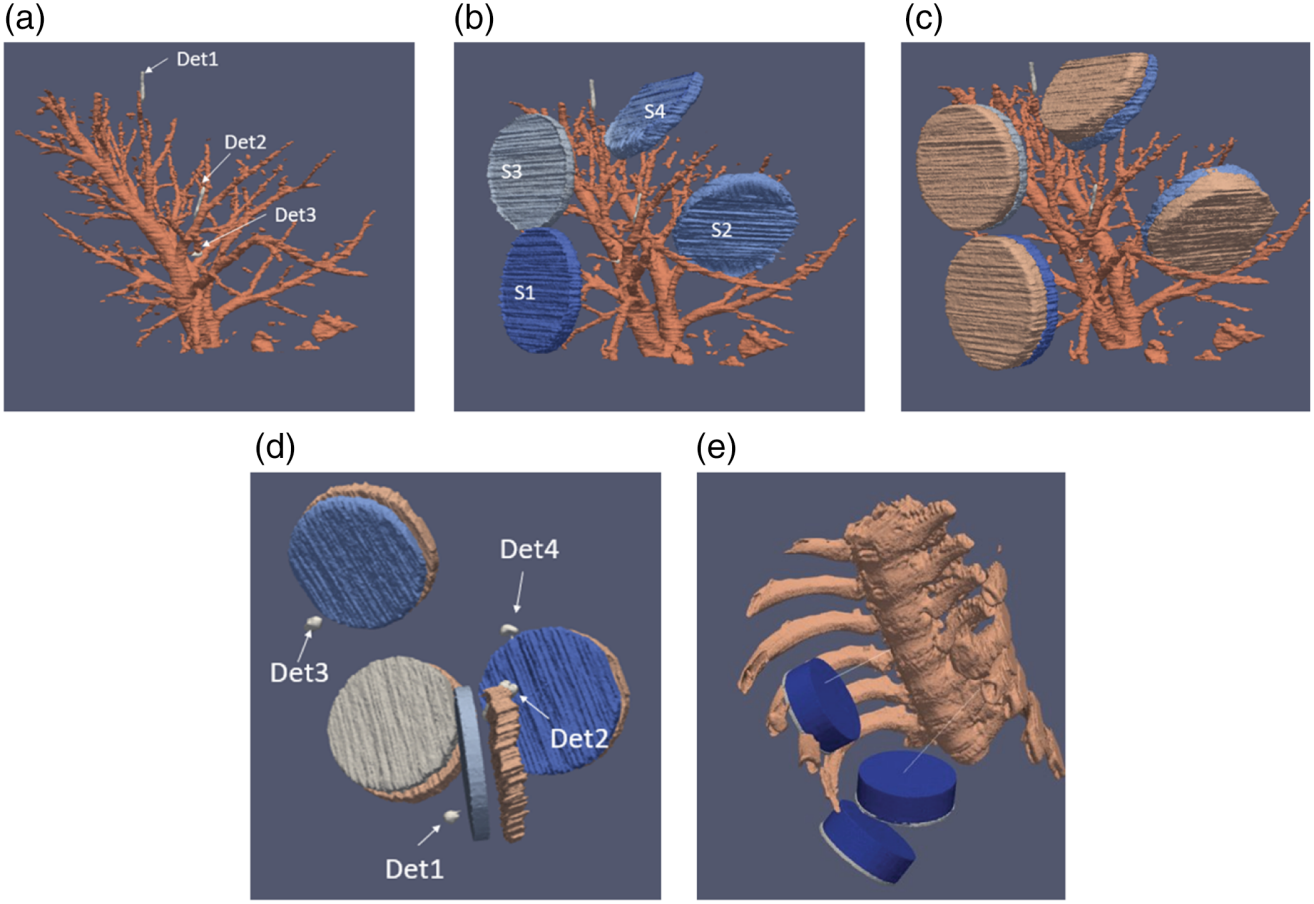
CT scans were segmented for the bronchial tree, lung parenchyma, sources, detectors, and surrounding rib cage. Panels show the progressive layers of the segmentation: (a) the bronchial tree and endobronchial light detectors, (b) the addition of the light sources, (c) the addition of non-reflective and non-emitting back of the light sources, (d) the detectors placed in front of each source as an internal control on max fluence outputted from each source, and (e) position sources in relation to the bony ribcage.
